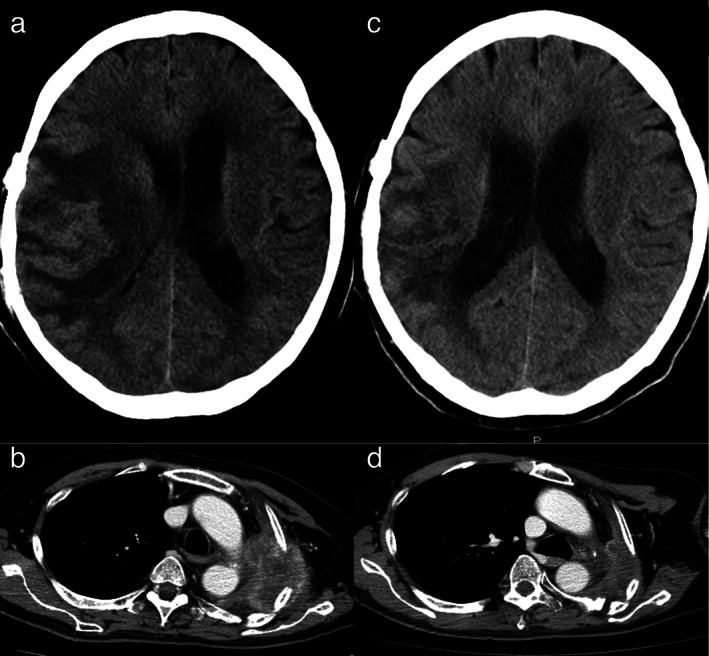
Brain and chest computed tomography (CT) before and after pembrolizumab administration. (a) Brain CT on Day 8 of the first pembrolizumab monotherapy cycle. Brain edema is markedly exacerbated in the right temporal lobe with midline shift. (b) Chest CT after two cycles of pembrolizumab. Chest wall tumors increased in size before initial pembrolizumab administration. (c) Brain CT after nine cycles of pembrolizumab. Brain edema has markedly decreased. (d) Chest CT after nine cycles of pembrolizumab. The chest wall tumor has markedly decreased in size
